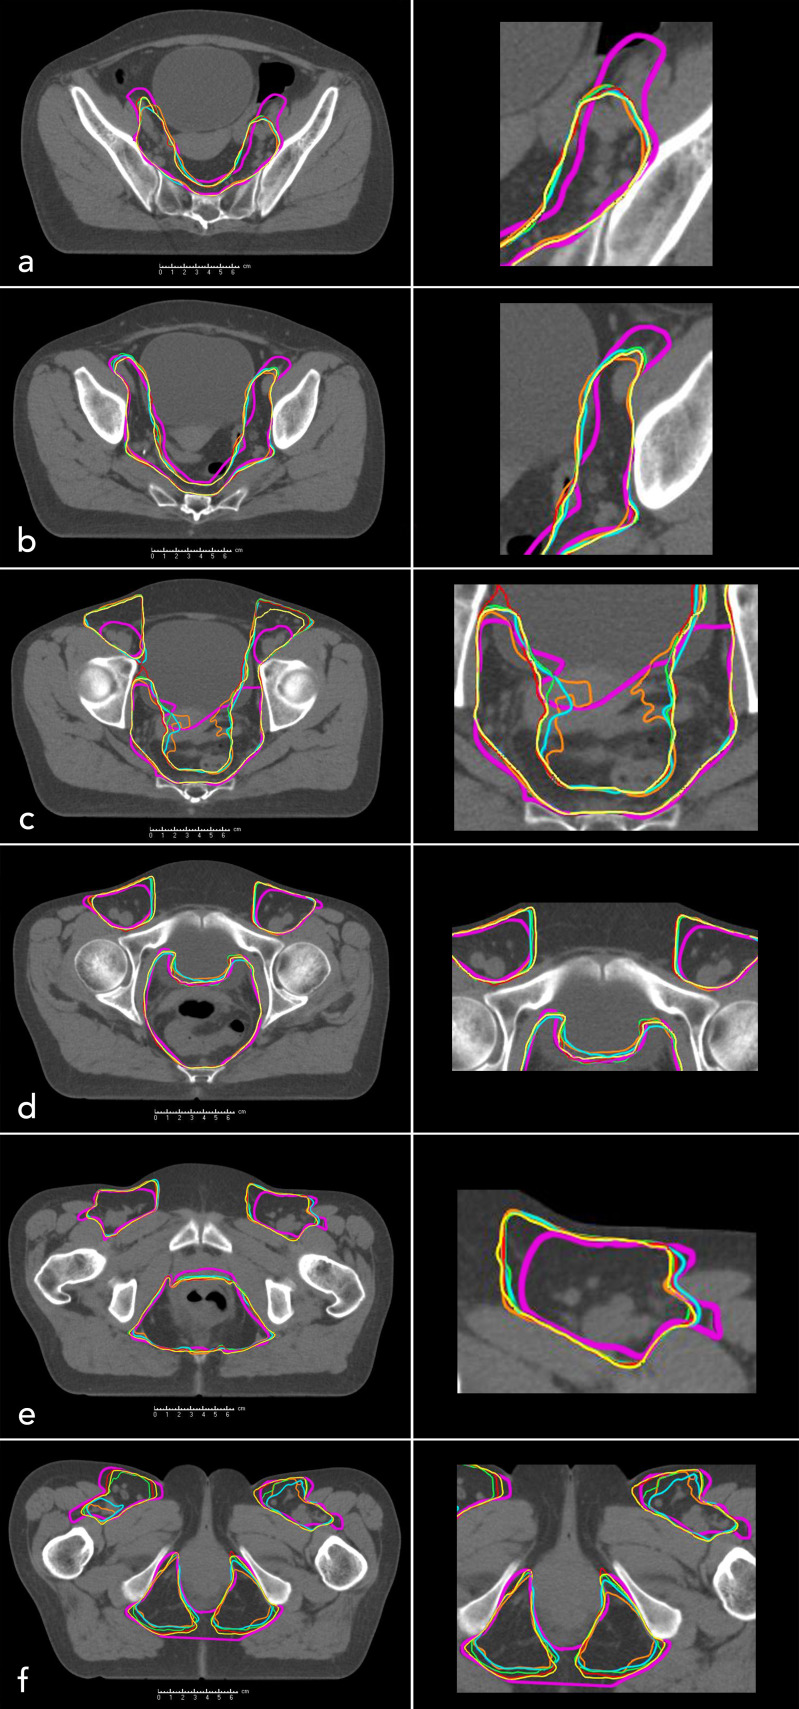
Manually contoured clinical target volume (mCTV, purple, patient 1) and automatically generated clinical target volumes for different atlas sizes: n = 10 (orange), n = 20 (blue), n = 30 (green), n = 40 (red), n = 50 (yellow). Axial CT slices of the pelvis are shown from cranial to caudal levels: (a–f).
